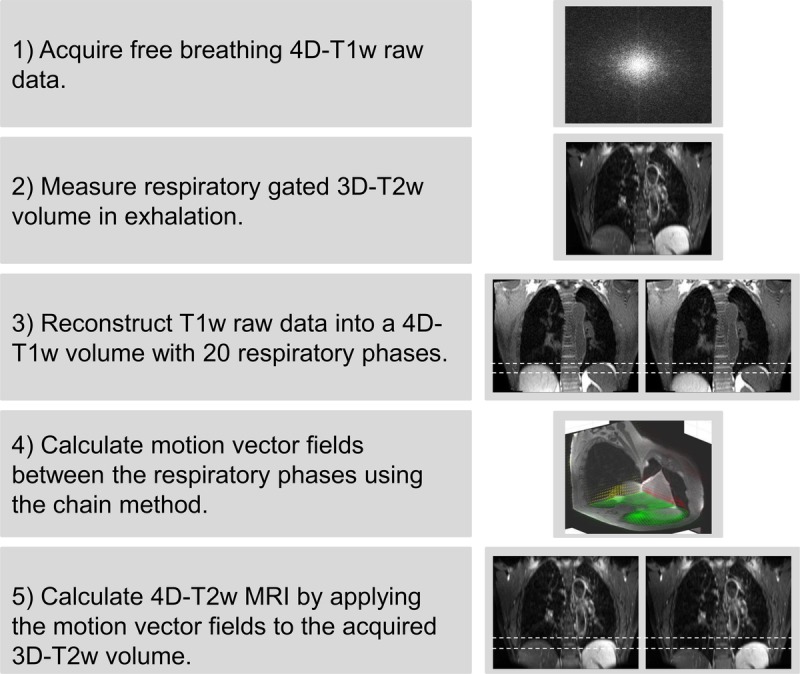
A visual overview of the motion vector field projection method. Note that the green, red, and yellow arrows in step 4 display the motion vector fields corresponding to the shown axial, coronal, and sagittal planes. Dashed white lines aid visualization of the diaphragm position on T1w and T2w MRI.
